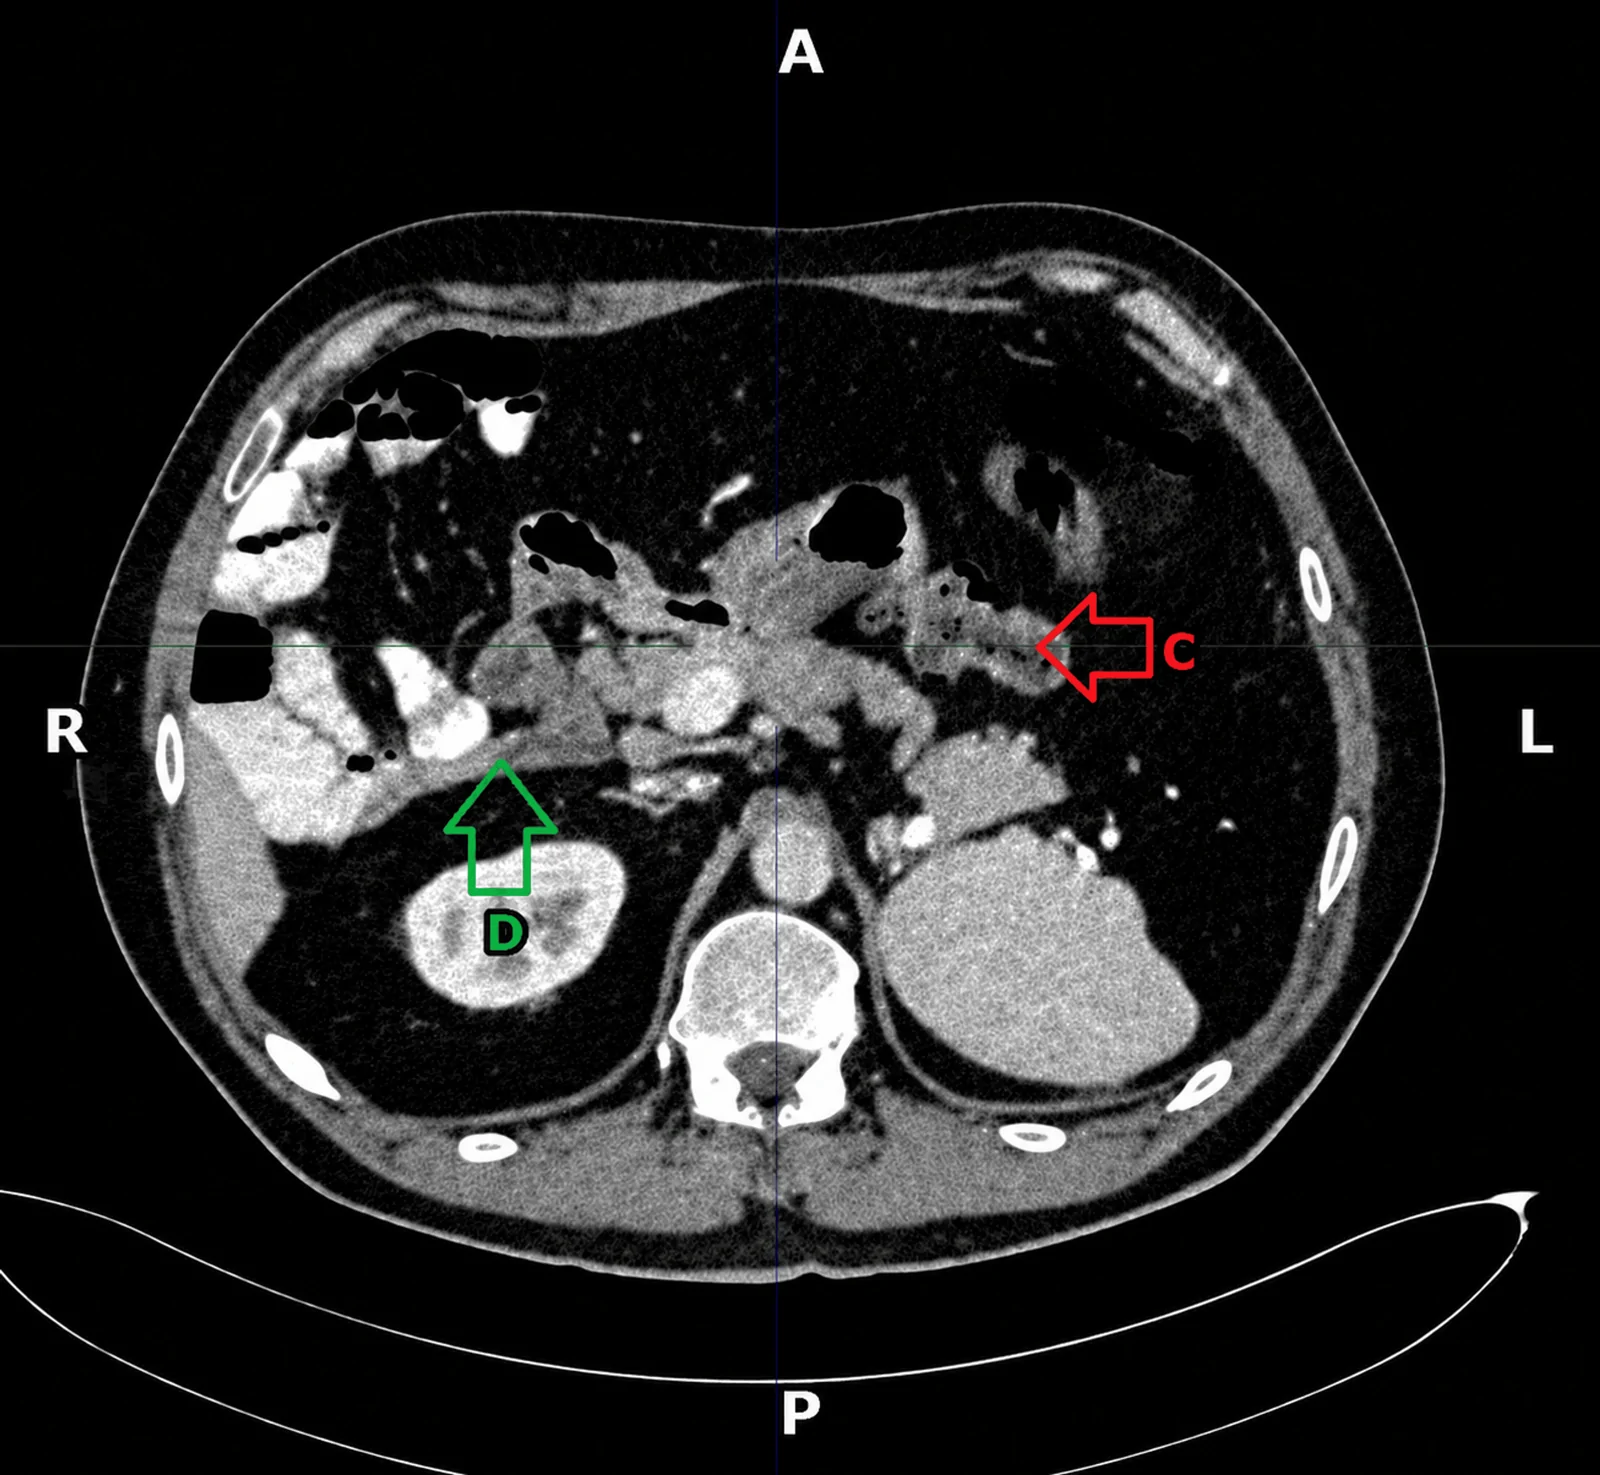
Axial contrast-enhanced CT image of patient 1 demonstrating right-sided predominance of the duodenum and jejunal loops, a midline course of the ascending colon, and a predominantly left-sided location of the remaining colon. The green arrow labeled D indicates the duodenum, and the red arrow labeled C indicates the colon. R, L, A, and P indicate the patient’s right, left, anterior, and posterior sides, respectively. CT: computed tomography
